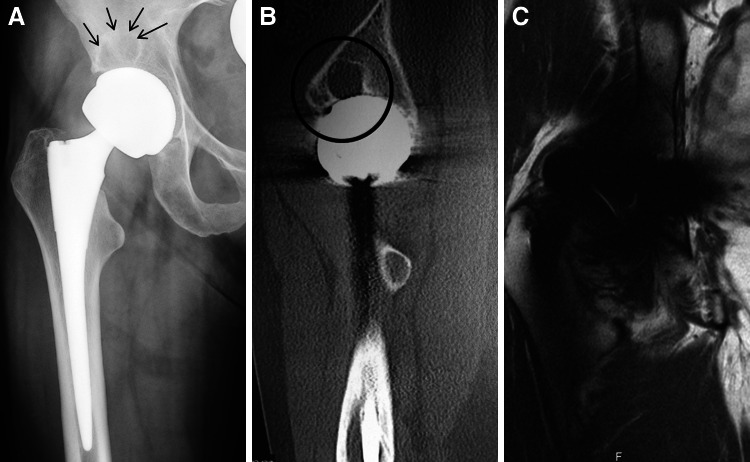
a Standardized anteroposterior pelvis radiographs demonstrating a Birmingham modular metal-on-metal total hip arthroplasty in a 58-year-old woman (patient 8) with periprosthetic lucency in DeLee zone 1 (*black arrows*). b Corresponding CT with metal artifact reduction showing periprosthetic osteolysis in the acetabular roof. c Corresponding MARS MRI with no evidence of acetabular osteolysis
